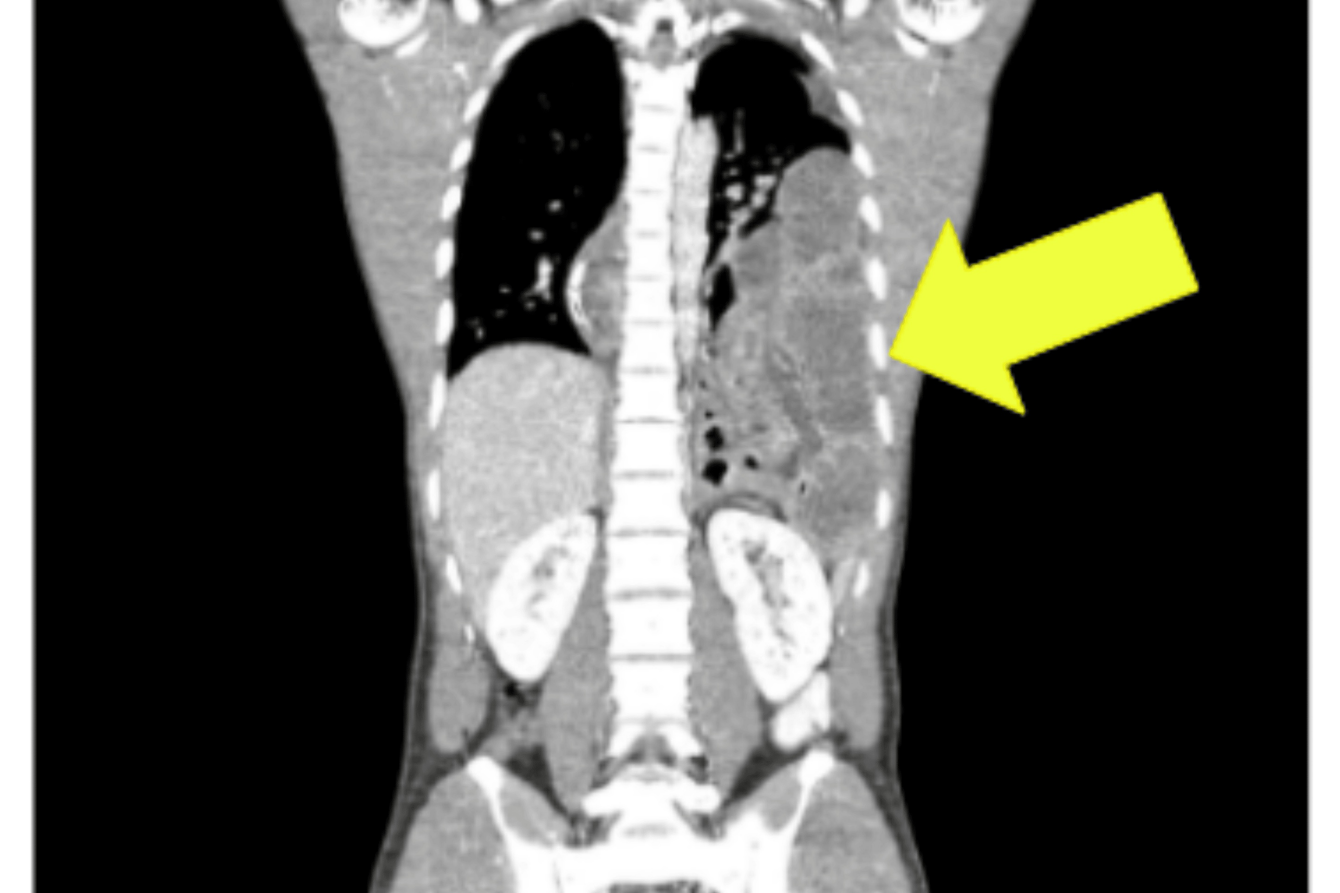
Computed Tomography (CT scan) Thorax, Abdomen and Pelvis - Coronal This accentuates the CT features of the left diaphragmatic hernia with signs suggestive of strangulation (yellow arrow).
